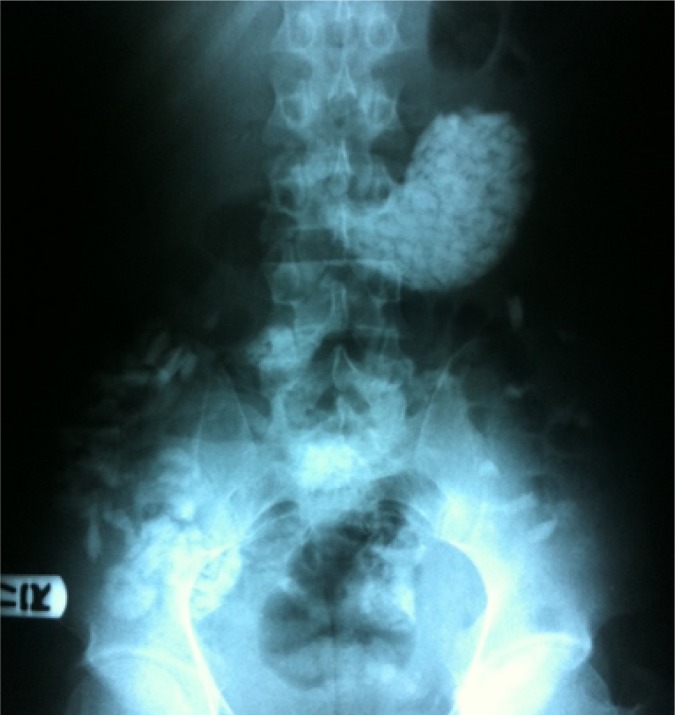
Upright abdominal X-ray showing a stomachfull of glass particles
